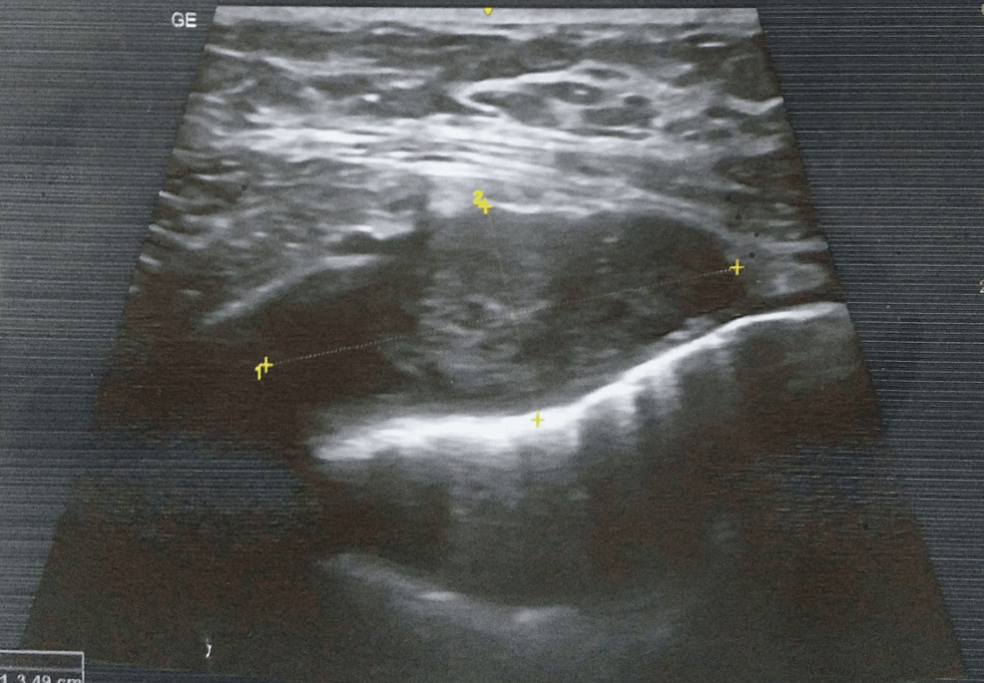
HRUSG of the right infraclavicular region was suggestive of intramuscular heterogenous collection HRUSG - high-resolution ultrasonography
